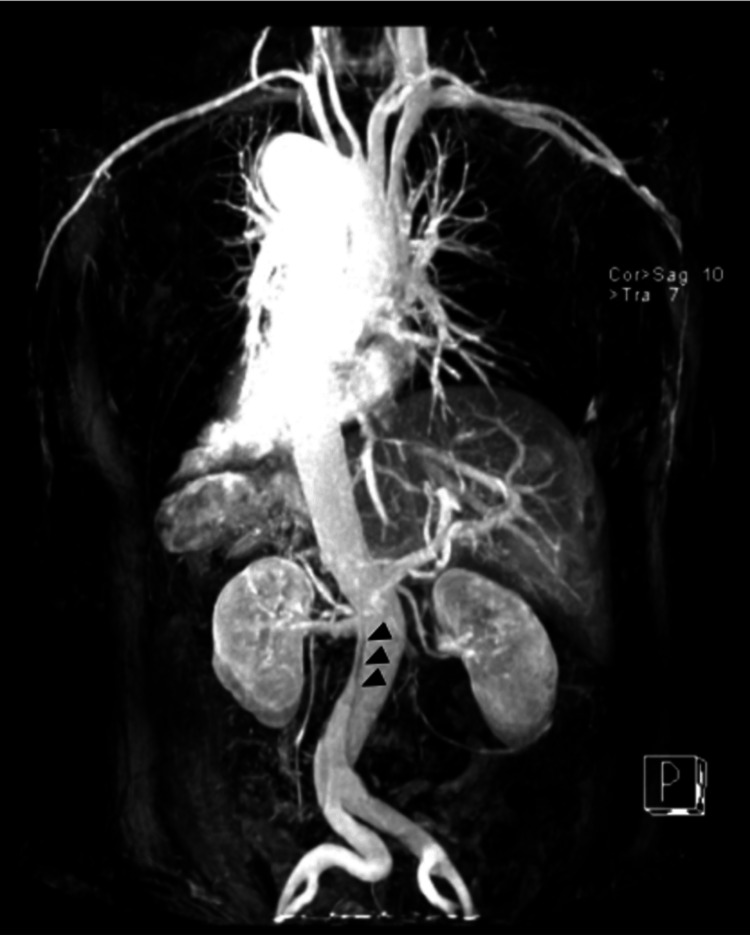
Three-dimensional MRI reconstruction of the aorta showing a flap (arrowhead).
